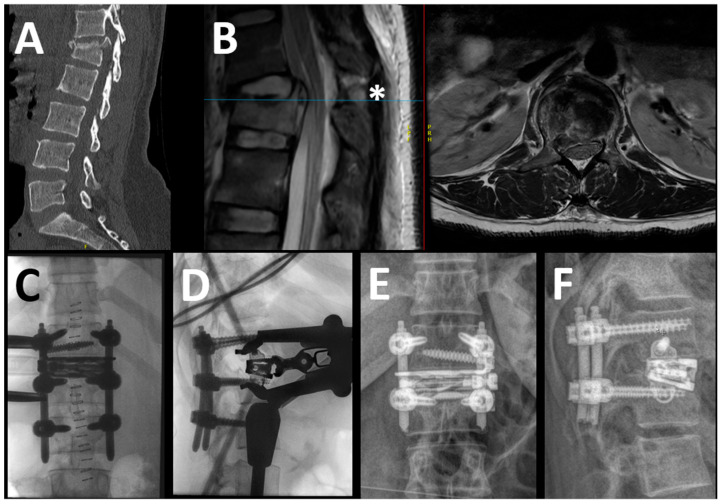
In this example, the case of a 39-year-old woman with a B2 injury at T12-L1 level (L1: A4) is reported. The patient presented without neurological deficits. In the upper row, the preoperative sagittal view of the CT scan (A) and the sagittal and axial T2 MRI (separated by the red line) (B) are shown, demonstrating the complete burst fracture of L1 along with the lesion of the posterior tension band, the blue line indicating the height of the axial imaging (*); in this case, there was no significant compression of the conus despite the slightly displaced posterior wall fragment. The intraoperative (C,D) and 1-year postoperative (E,F) images are shown in the lower row. In this case, we placed monoaxial screws percutaneously (no need for canal decompression) to effectively restore the alignment and performed the LLIF in the same anesthesia (=one-staged, temporary bisegmental). Notice the position of the screws in L1, which act as additional support for the expandable spacer.
